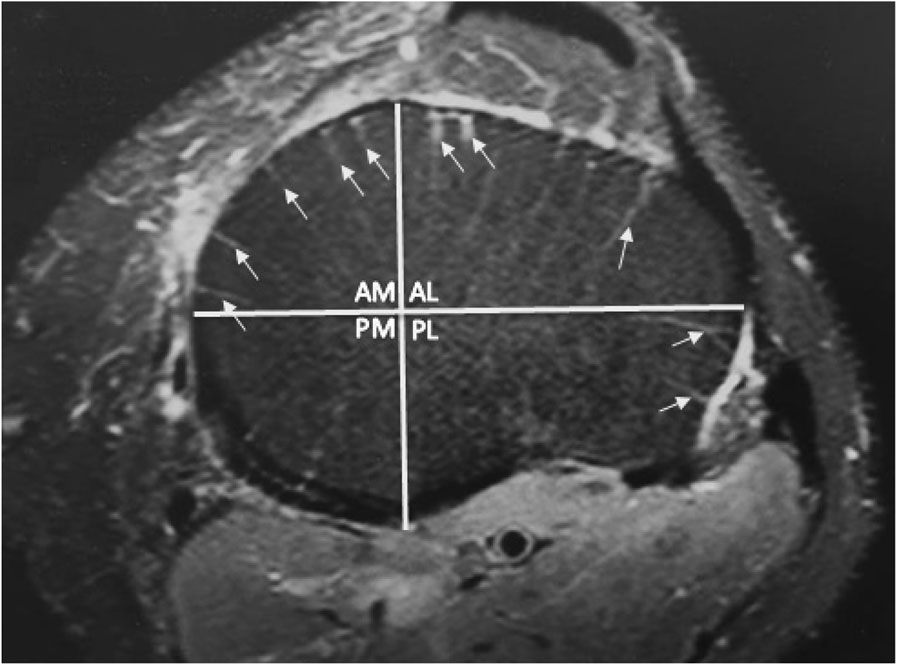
Example of quadrant division and scoring. The same left knee level 3 MRI slice seen in Fig. 1 with scoring marks. This slice scored 5, 0, 2, and 3 for anteromedial, posteromedial, posterolateral, and anterolateral quadrants
